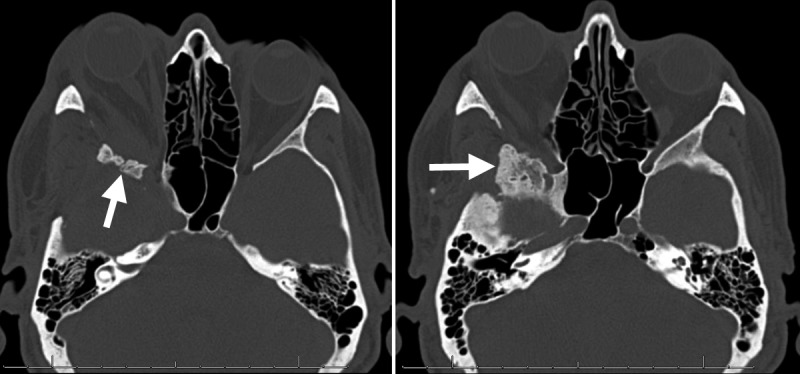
Computed tomography of the orbit Axial cuts showing recurrent bony growth of the sphenoid wings, lateral sphenoid sinus wall, and middle cranial fossa floor (arrows).
